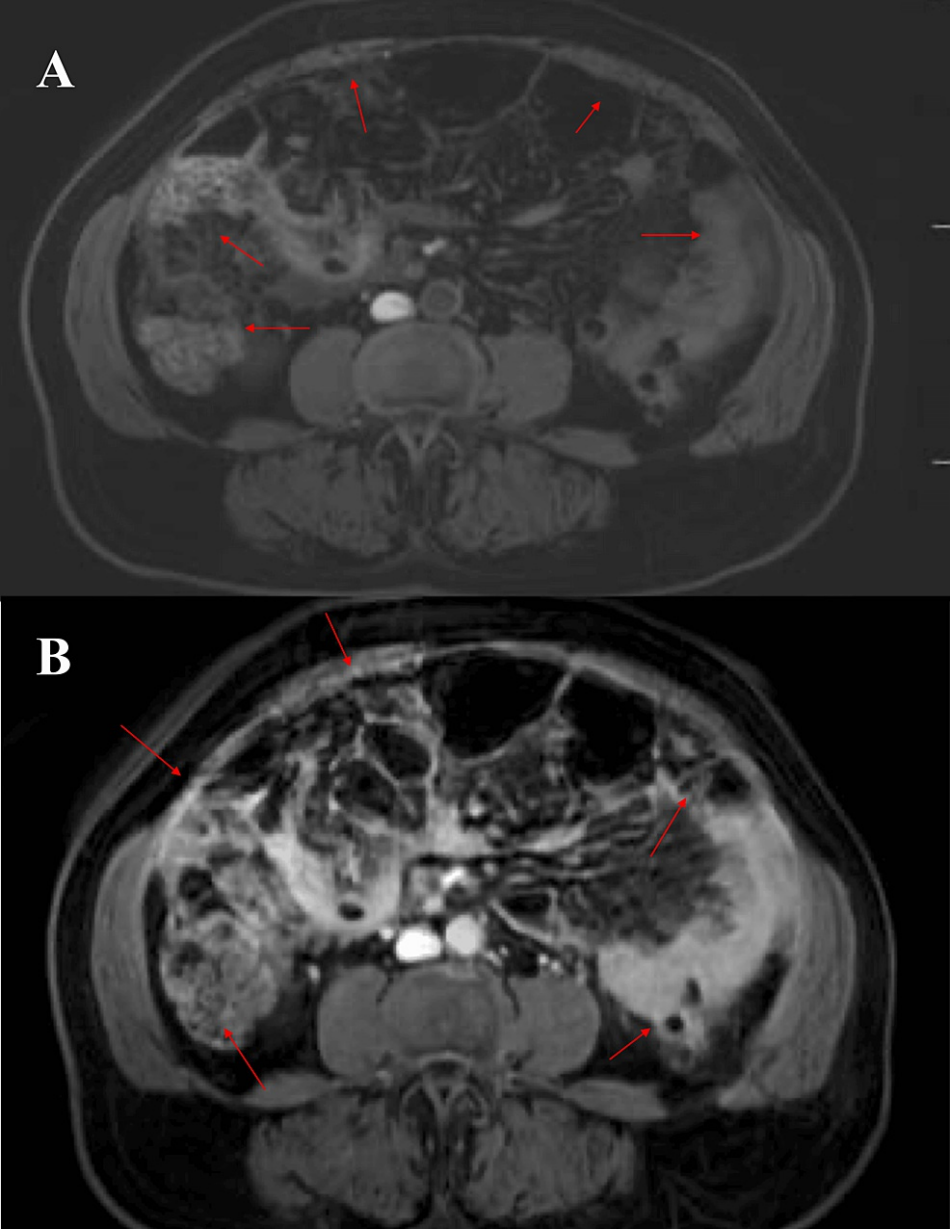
Abdominal T1-weighted axial view MRI without contrast (A) and T2-weighted axial view MRI with contrast (B). Appendiceal and ileocecal thickening with abdominal and pelvic ascites, peritoneal thickening, and omental nodularity (arrows).
